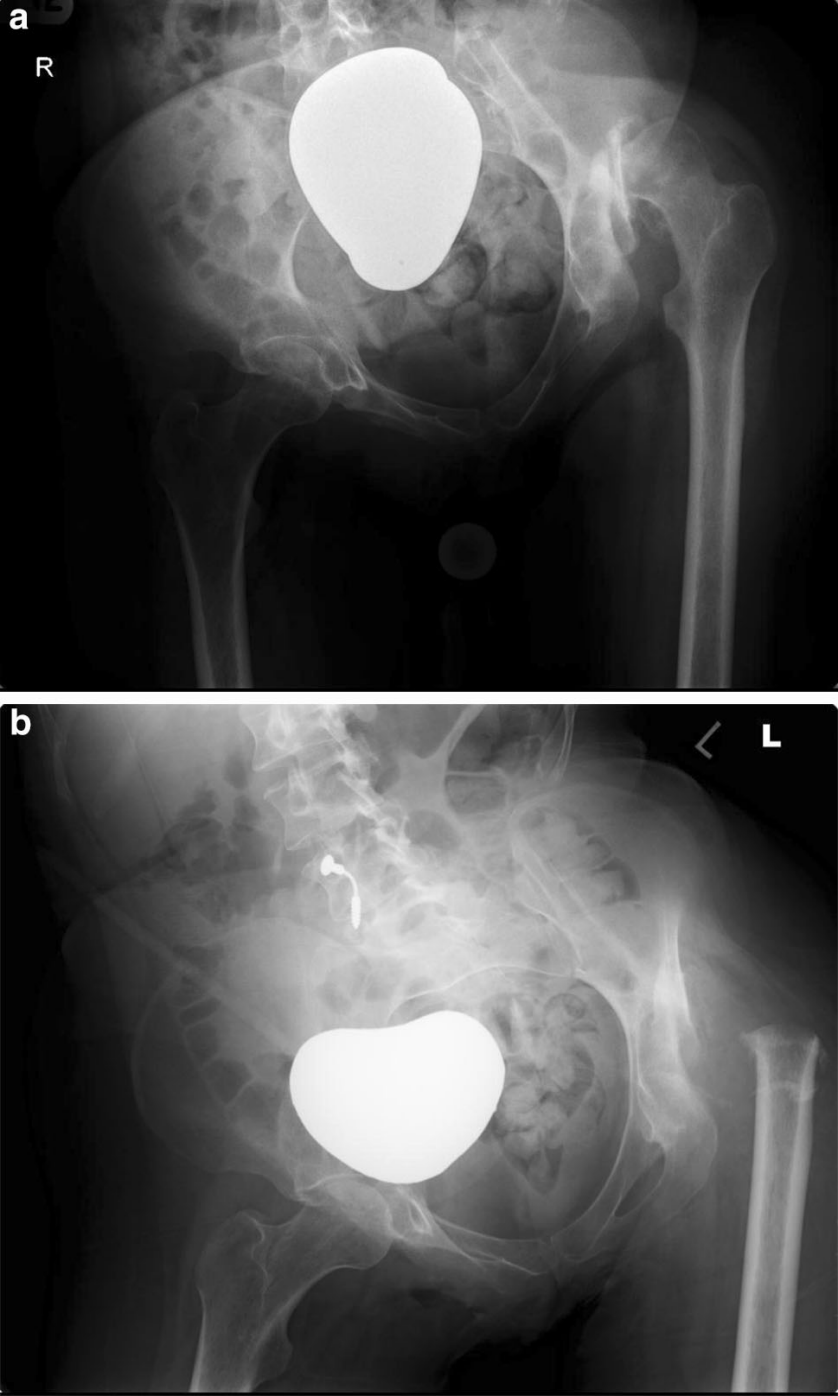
Pre- (a) and post-operative (b) anteroposterior pelvic radiographs of a 32-year-old female with a painful dislocated left hip and secondary osteoarthritis undergoing a proximal femoral excision
